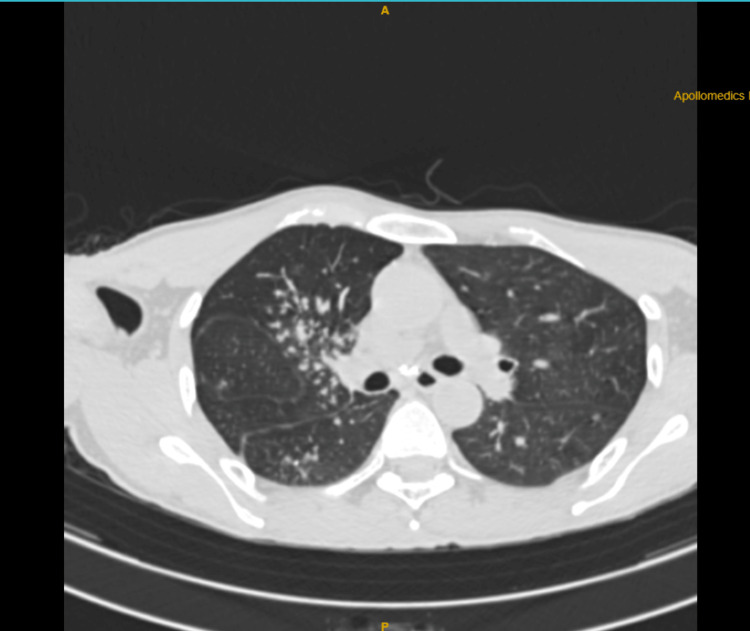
CT of the neck and thorax: tree-in-bud appearance in the right upper lobe of the lung.
